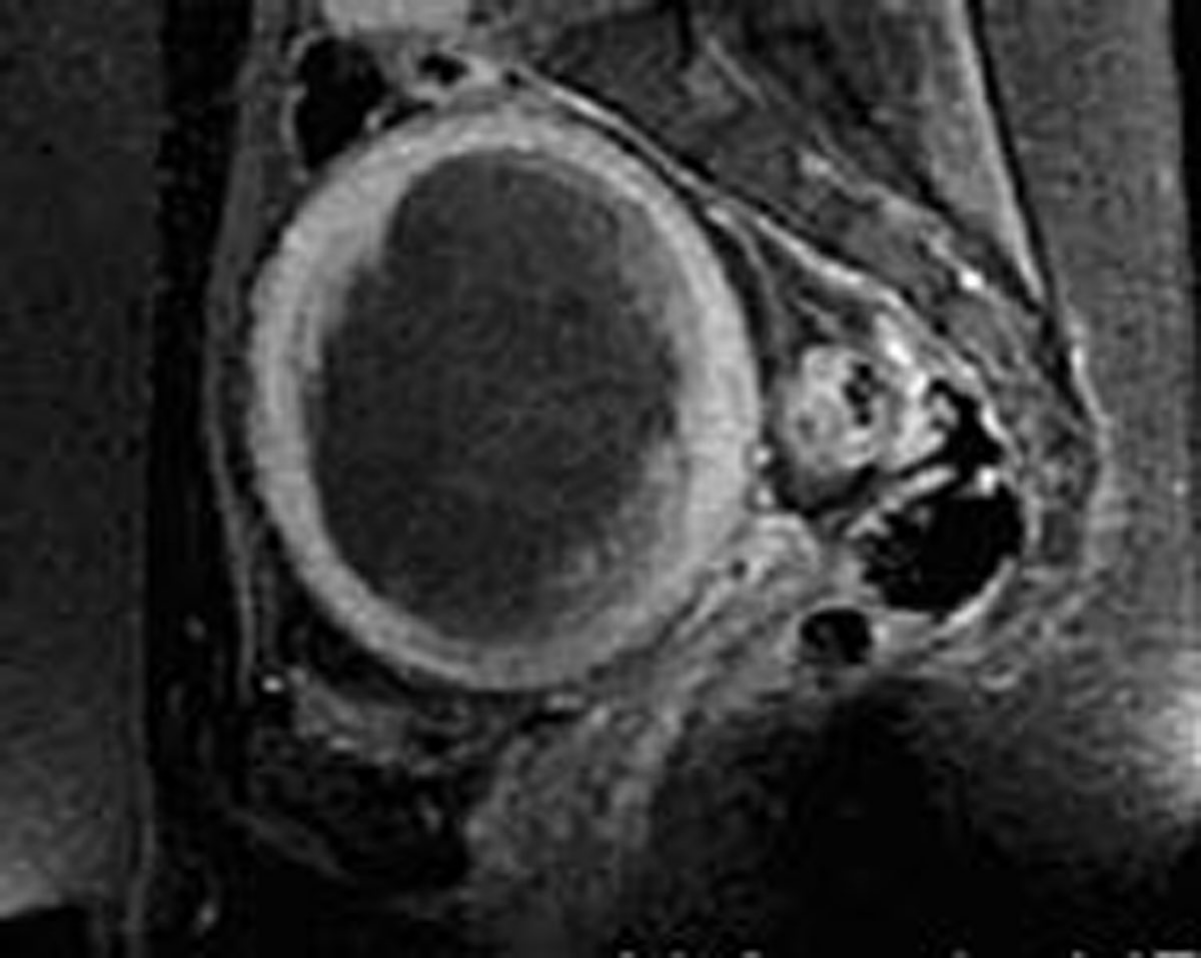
Sagittal post-contrast T1-weighted image following MRgFUS treatment demonstrates a non-perfused volume of 85%.
